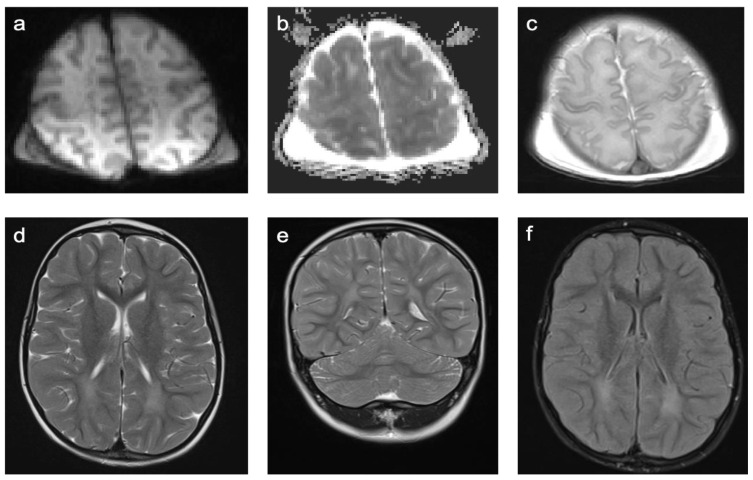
Representative case “Non-genetic hypoxic and ischemic insults”: term-birth asphyxia. DWI/ADC (a,b), T2W (c–e), FLAIR (f) sequences. Neonatal resuscitation after severe term-birth (40 weeks) asphyxia in a three-day-old girl. (a–c). Treatment with therapeutic hypothermia. MRI shows predominantly white matter injury as one of the patterns identified in term-birth asphyxia [20]. The same girl examined again at the age of four years (d–f). MRI shows periventricular signal hyperintensities in T2W/FLAIR sequences.
